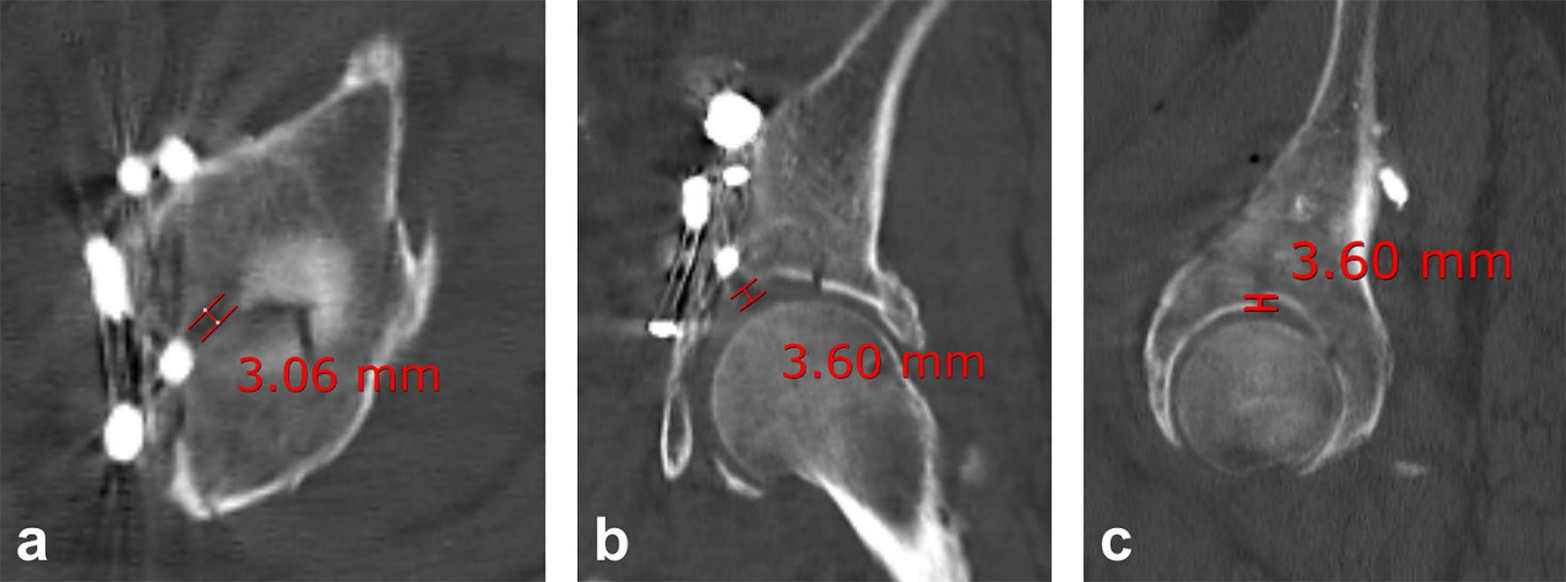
Evaluation of quality of reduction. (a) Gap and (b and c) step displacement in the weight-bearing dome was evaluated based on postoperative computed tomography with (a) axial, (b) coronal, and (c) sagittal planes.
